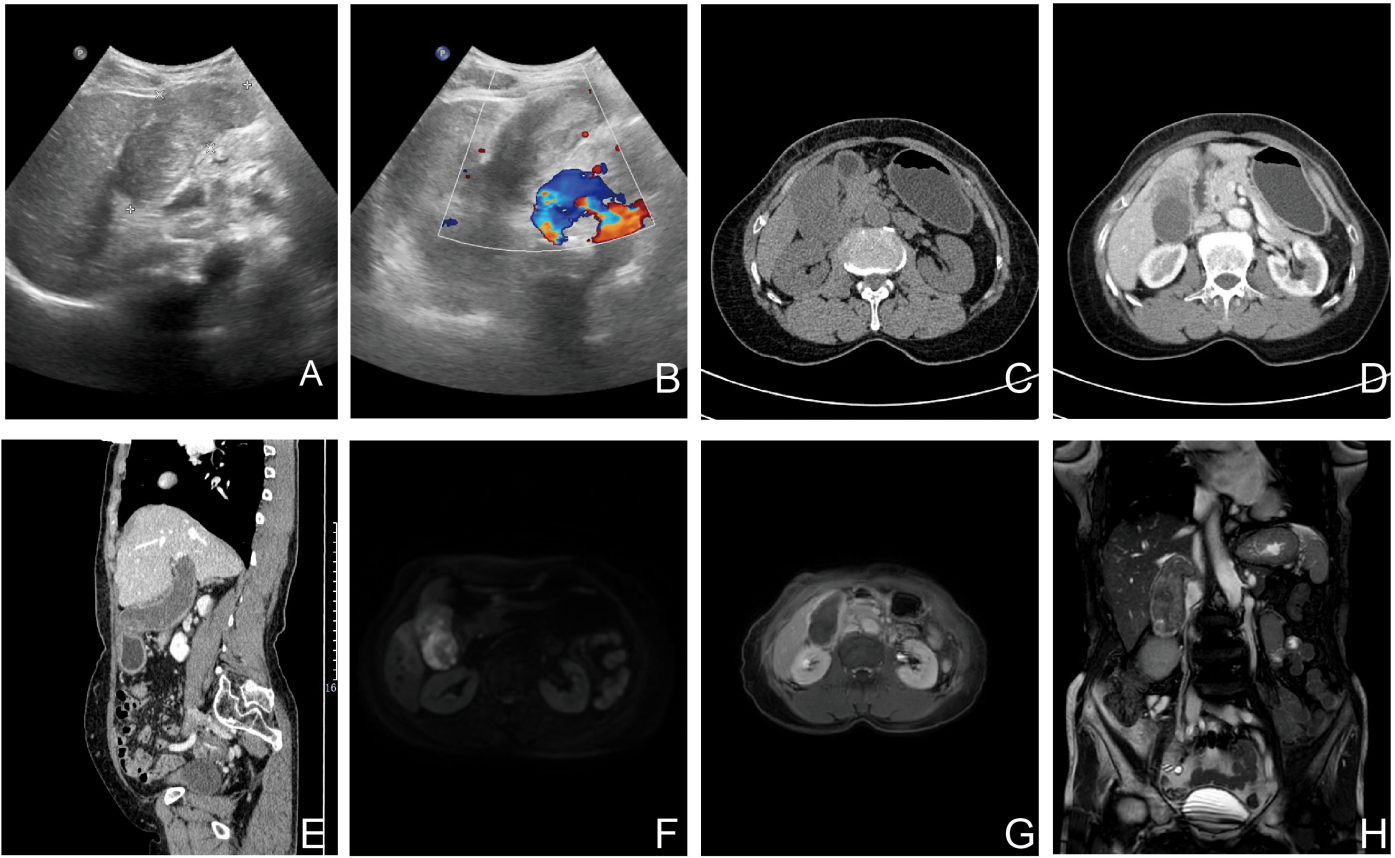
Preoperative imaging of the GBCS (Ultrasound, CT and MRI). Color doppler ultrasound images: (A, B) The images show a hypoechoic mass with well-defined margins. The Doppler signals (red and blue) demonstrate the vascular distribution around the lesion. These findings have some value in the preoperative differentiation between benign and malignant lesions but are non-specific. (C-E) Unenhanced and contrast-enhanced CT demonstrate an enlarged gallbladder with diffuse wall thickening and enhancement upon contrast administration. (F-H) Diffusion-weighted imaging (DWI), contrast-enhanced T1-weighted, and T2-weighted coronal images show gallbladder wall thickening accompanied by enhancement and restricted diffusion. These findings are highly suggestive of malignancy.
